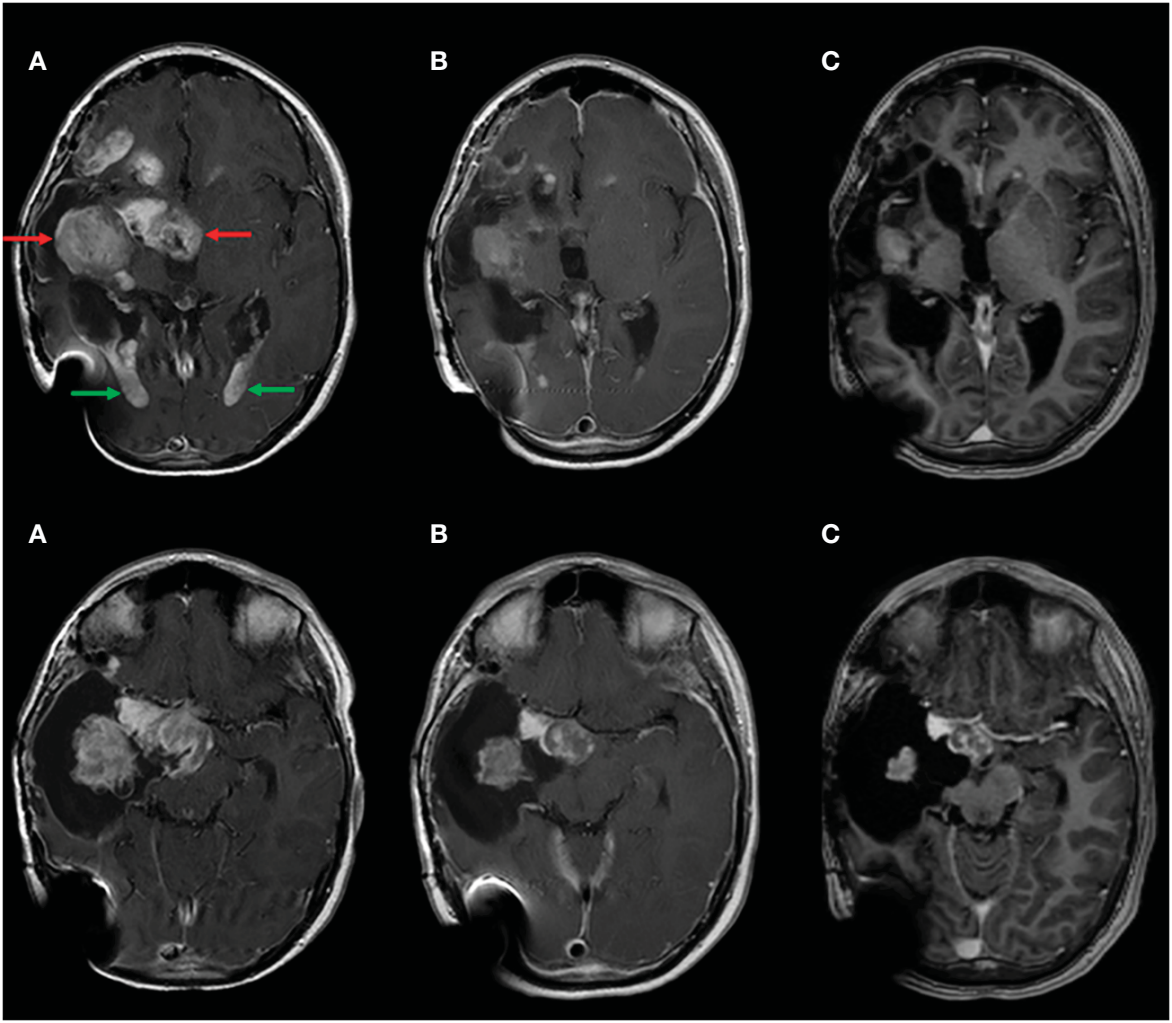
Contrast material-enhanced T1-weighted MR image shows reduction of the solid part of the main tumor (red arrows) as well as the intraventricular enhancing masses (green arrows). (A) Pre-treatment with trametinib; (B) before reducing trametinib dose; (C) end of treatment.
